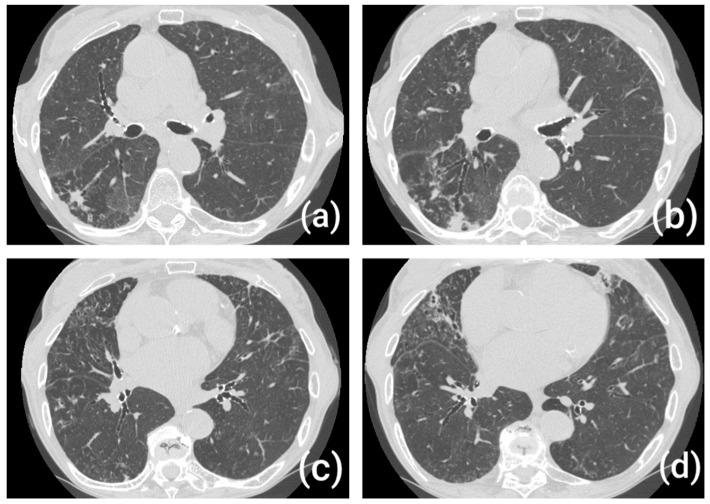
(a–d) HRCT scans with tubular and predominant basal bronchiectasis in AATD patient. Abbreviations: HRCT, high-resolution computed tomography.
